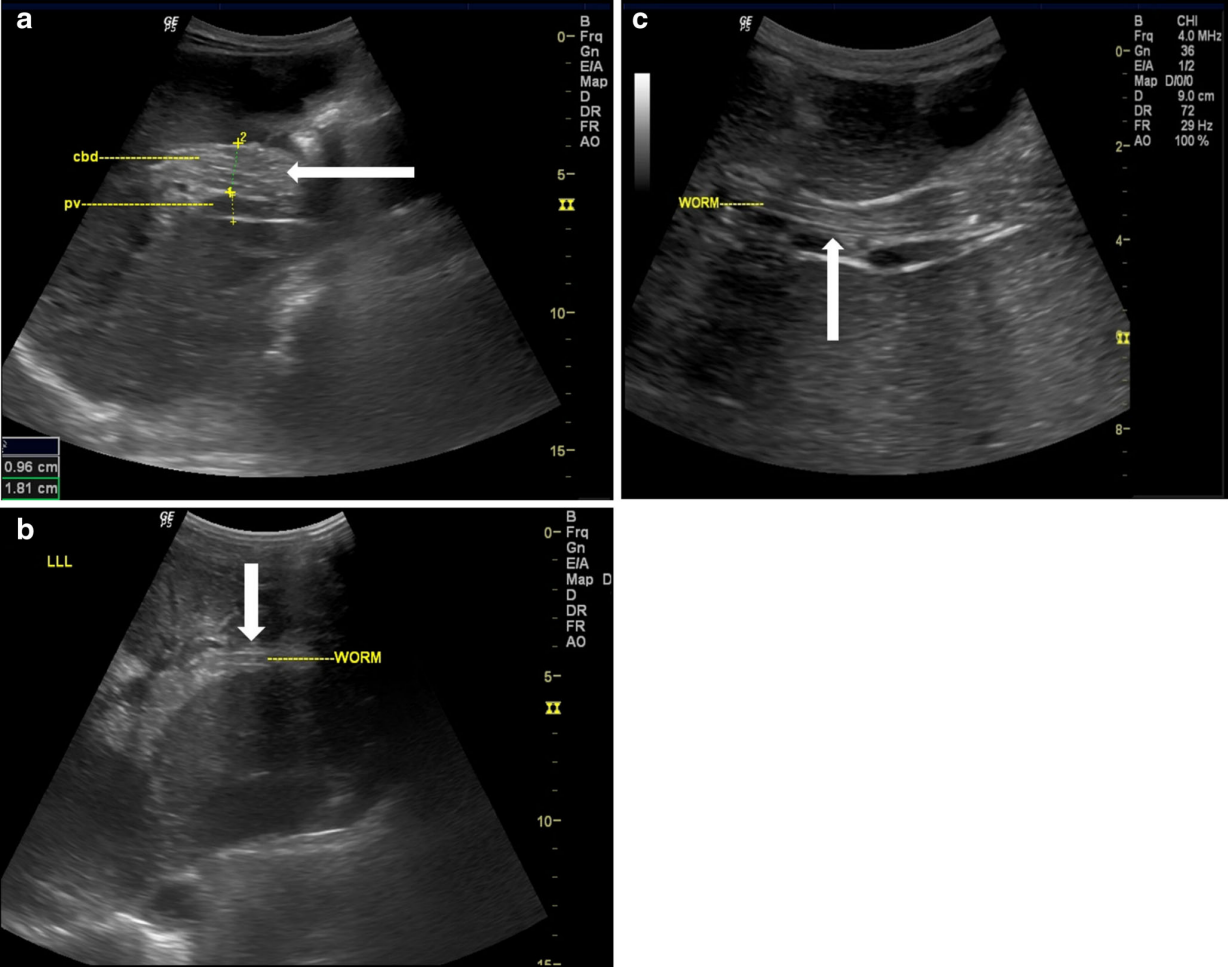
A 14-year-old female with acute biliary colic. (a) Multiple ascaris in CBD, the spaghetti sign, CBD is filled with multiple worms (*long thick white arrow*), (b) intra hepatic duct of the left lobe of liver showing a double linear echogenic wall indicating ascariasis (*white arrow*) and (c) following antihelminthic medication, sonography was repeated the next day showing reduction in the number of worms. Note double echogenic walls (*strip sign*) of CBD round worm (*white arrow*)
